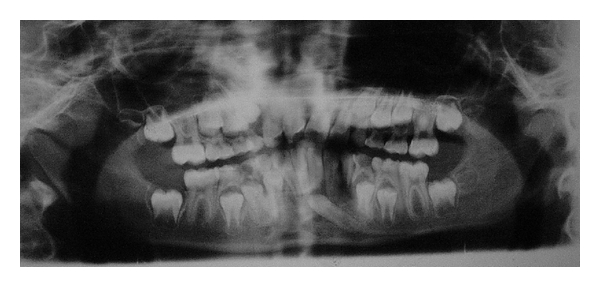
Panoramic radiograph showing tooth buds of all permanent teeth.
